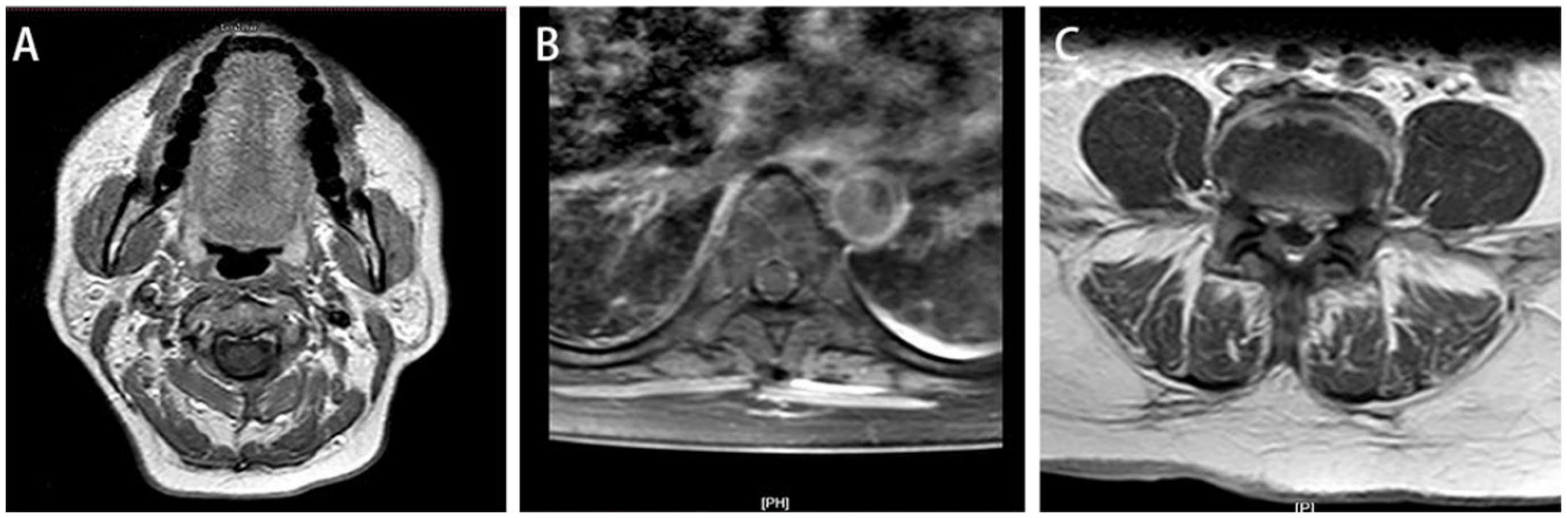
At 2-month follow-up, sagittal T1-weighted (A) and T2-weighted image (B) and showed the lumbar disc herniation was still present, and the epidural space in the posterior lumbar and anterior sacrococcygeal segments was wider than before, and the enhancement of the dural sac improved after enhancement compared with before. Axial T1-enhanced image (C) showed the previously large range of irregular non-enhanced areas has decreased compared to Figure 1F.
